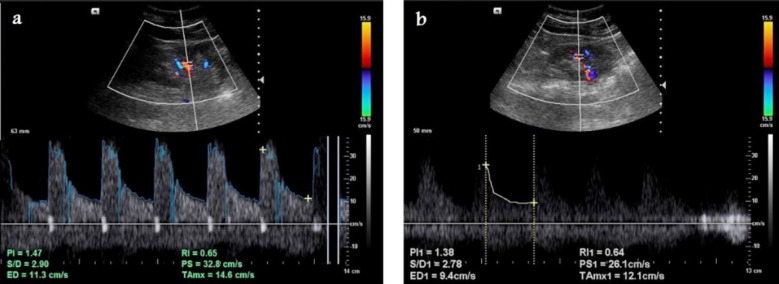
Selected spectral Doppler ultrasound images of the same 50-year-old female diabetic patient referred for follow up ultrasound imaging show Doppler measurement of RI (a) automatically, and (b) manually that was slightly higher than normal.
